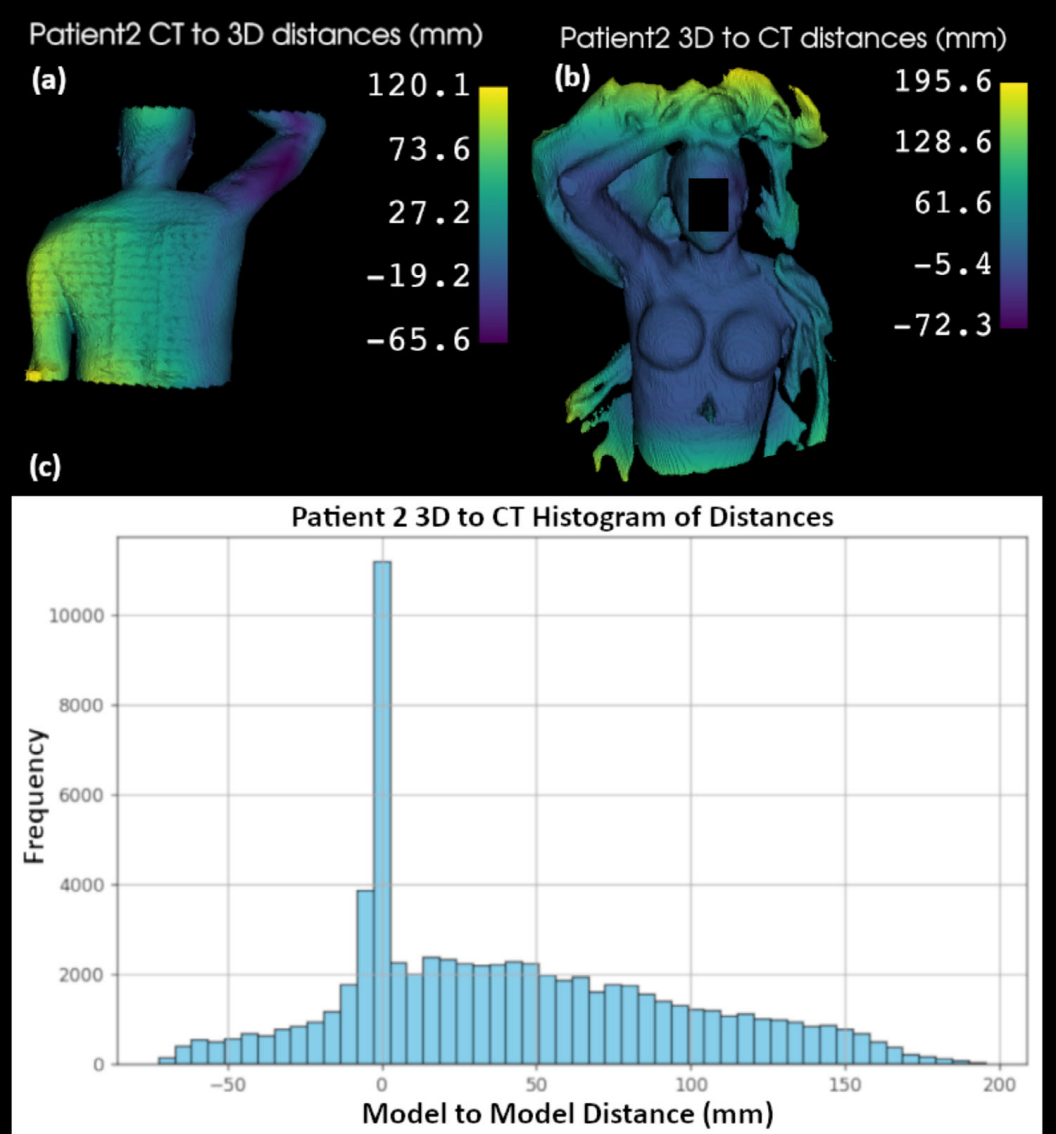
Model to model distance maps and histogram for patient 2, showing the greatest disagreement in areas where the 3D and CT FOVs differ. (a) Patient 2 CT to 3D distances showing the hidden posterior aspect of the patient that could not be 3D scanned, (b) Patient 2 3D to CT distances showing the extended data and immobilization information that does not correlate with the acquired CT surface, (c) Histogram of all the distances calculated for the 3D to CT evaluation.
